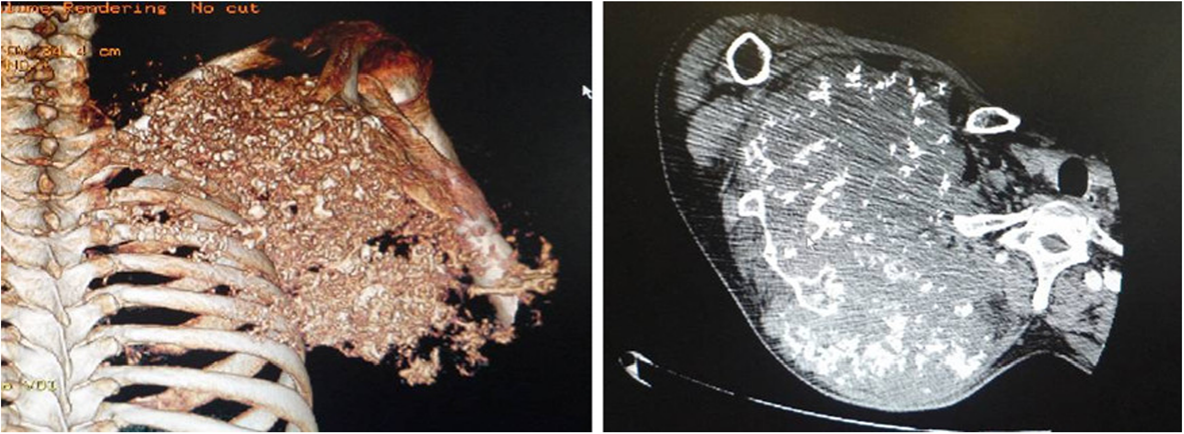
The computer tomography (CT) image of the right shoulder showing the tumor in the right scapula before surgery.
